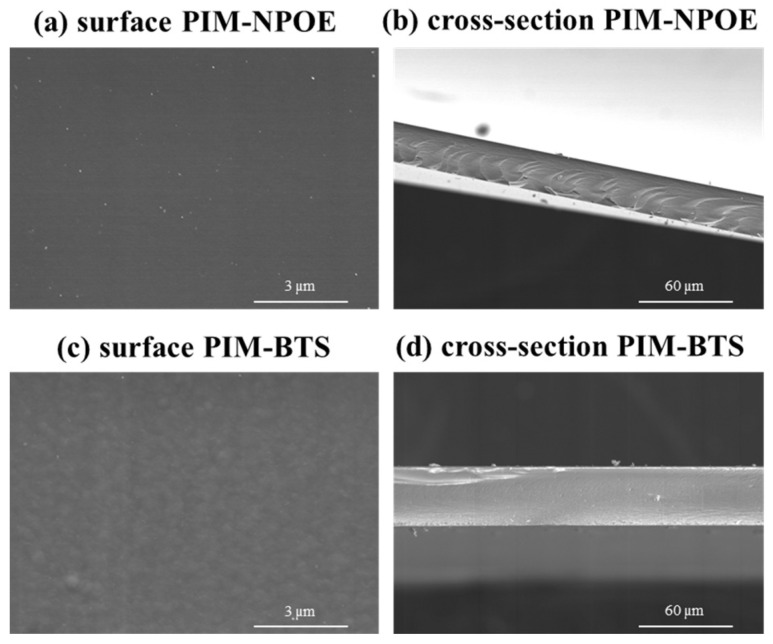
SEM images of CTA-based PIMs (50% CTA, 30% TOMATS, and 20% plasticizer (a,b) NPOE or (c,d) BTS).
